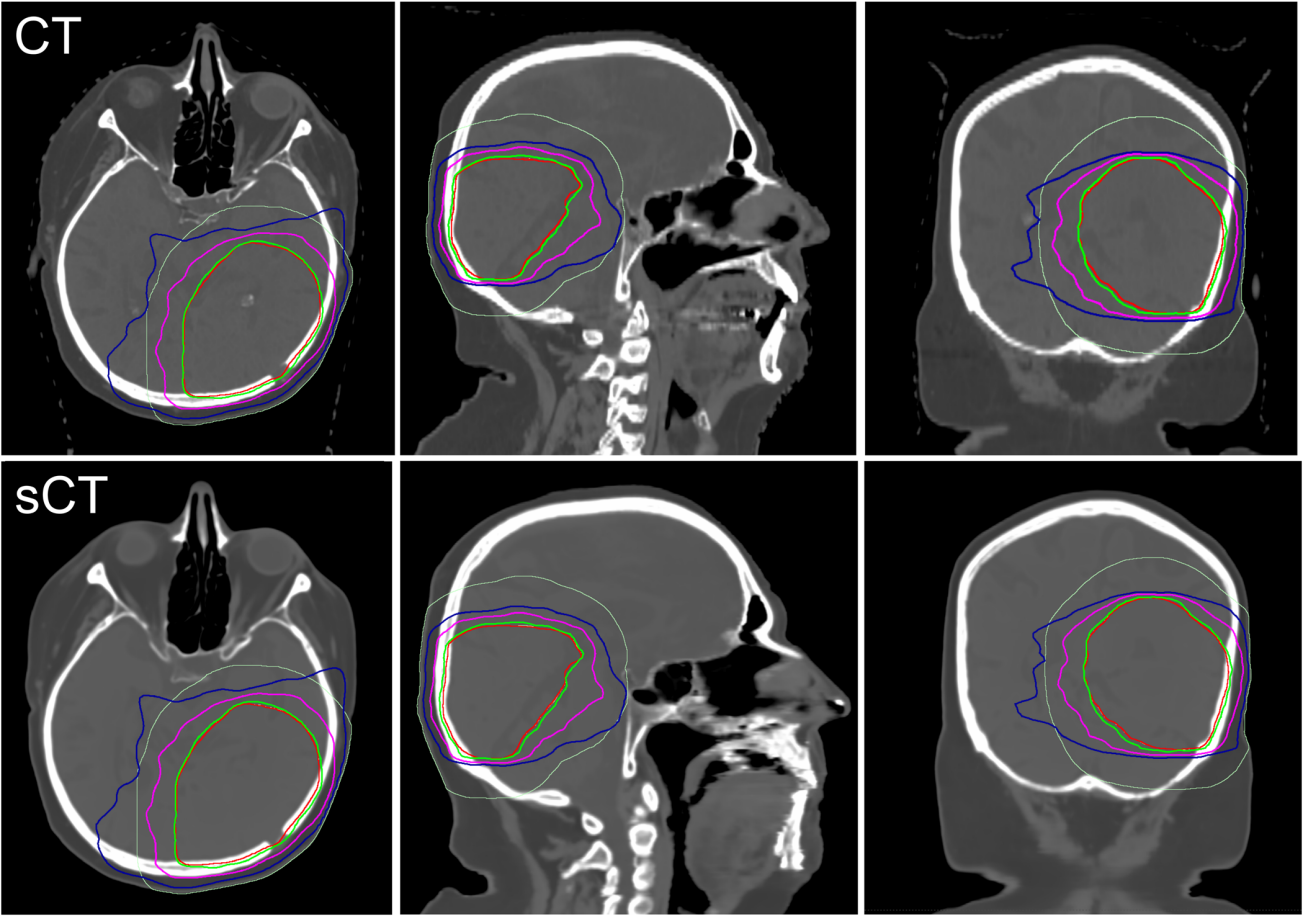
A case example of CT versus sCT image quality of a glioma patient with identical windowing parameters, showing the PTV (red) and the 2 cm NT (light green) structure outlines. Relative isodose contours of 95% (green), 70% (magenta), and 50% (blue) are visible. CT, computed tomography; NT, normal tissue; PTV, planning target volume; sCT, synthetic computed tomography.
